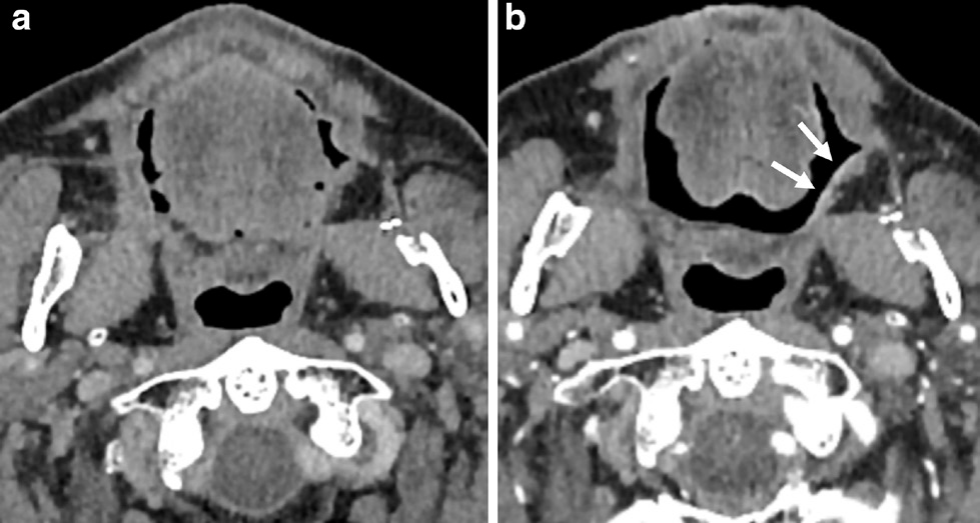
a,b. Post-treatment surveillance CT images of a patient with OSCC located primarily in the left mouth floor obtained 18 months (a) and 24 months (b) after resection and adjuvant radiotherapy. There is new enhancement on the surface of the radialis flap reconstruction (b, indicated by white arrows). The initial assignment of NI-RADS category 2a for the primary site was downgraded to category 1 by the supervising radiologist. There was no evidence of cancer recurrence in clinical inspection and subsequent surveillance CT. OSCC, oral squamous cell carcinoma
